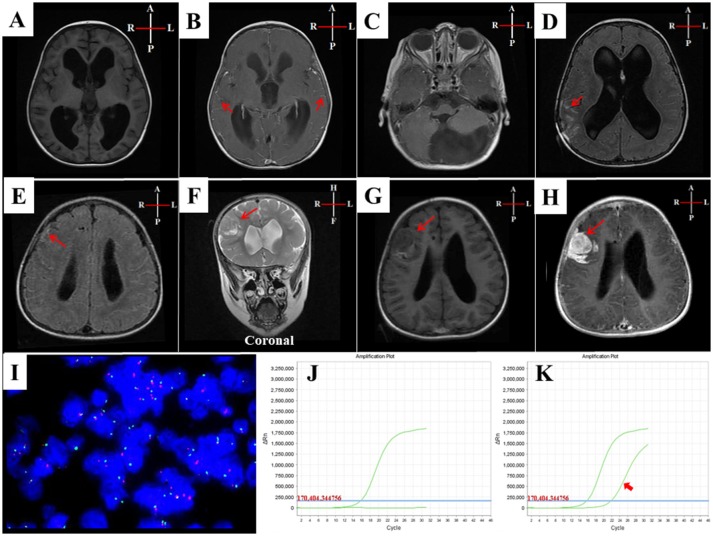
MRI and cytogenetic characteristics of case-I. (A–H) MRI of case-I: (A) T1W-axial-pre-contrast and (B) T1W-axial post-contrast, showed hydrocephalus of third and lateral ventricles with diffuse leptomeningeal enhancements (arrows in B). (C) T1W-axial-post-contrast at posterior fossa level, showed a normal fourth ventricle with large arachnoid cyst at cisterna magna. (D) T1W-axial-post-contrast, 3 months and (E) 6 months later, showed evidence of ventriculoperitoneal shunt with persistence of the hydrocephalus and leptomeningeal enhancement and looked thick at the right parietal lobe in D and right frontal lobe in E (arrowed). (F) T2W-coronal image (G) T1W-axial image after 9 months, showed 3 cm extra-axial dural based intermediate signal lesion at the right frontal lobe surrounded by vasogenic edema causing mass effect on the right lateral ventricle and showed an intense enhancement in (H) T1W-axial-post-contrast image. (I) FISH analysis revealed a lack of allelic deletion of P53. (J) Genetic analysis of ARMS-PCR results detected no *BRAF*^V600E^ mutation. (K)
